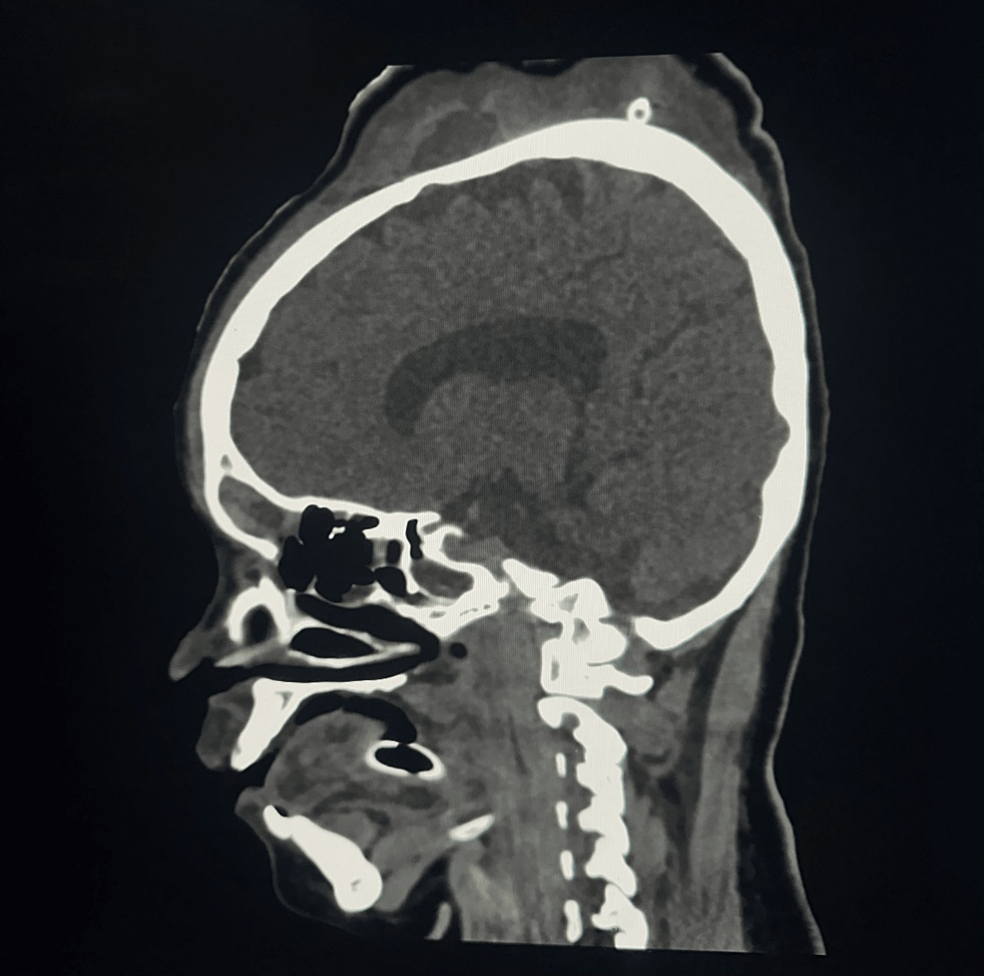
Computed tomography showing minimal residual hematoma in the extracalvarial space with a negative suction drain
